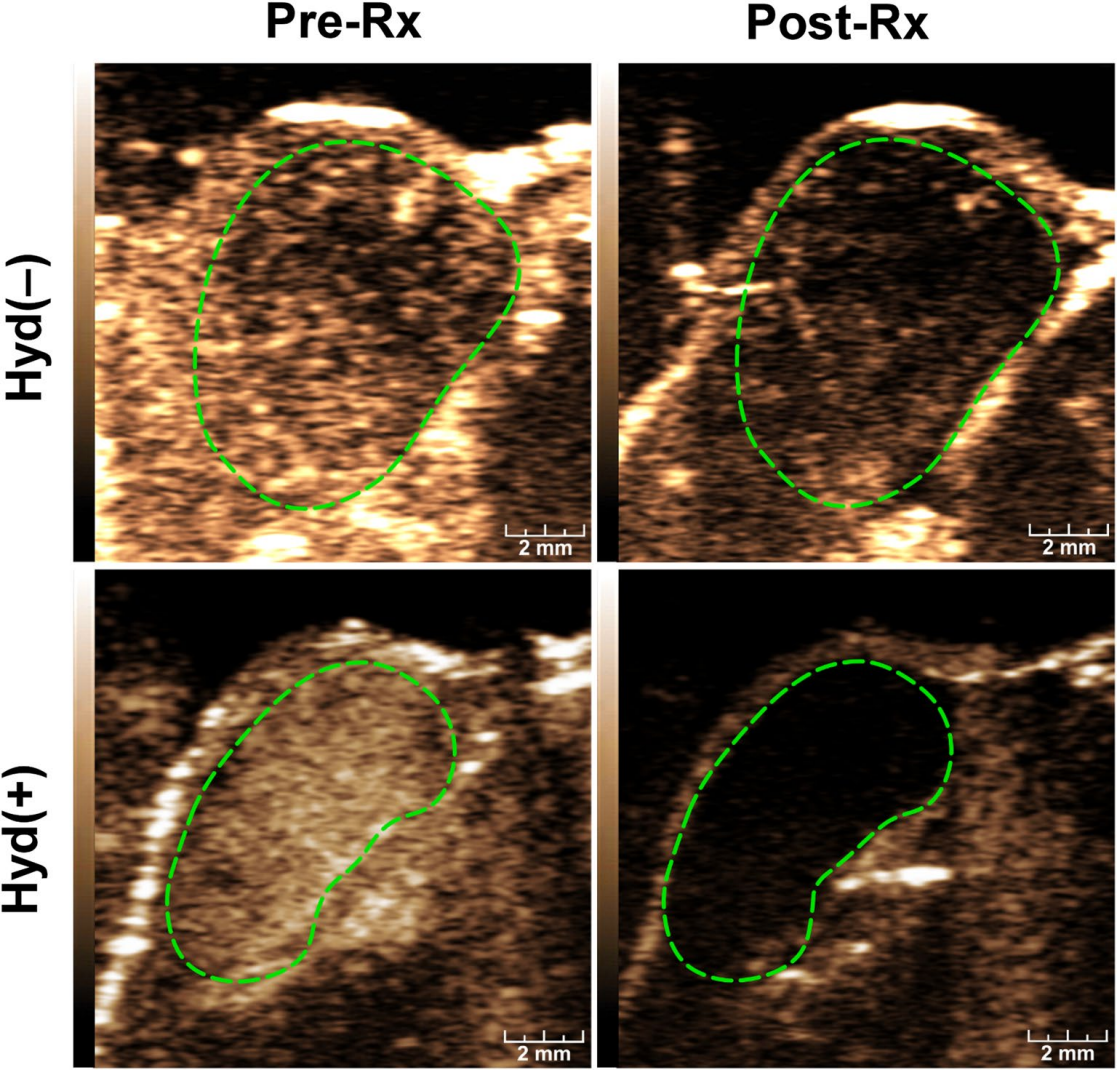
Effects of combined ultrasound and hydralazine treatments on HCC blood flow. Non-linear contrast-enhanced (NLC) images were acquired prior to (Pre-Rx) and following (Post-Rx) ultrasound treatment with [Hyd(+)] or without [Hyd(−)] administration of 5 mg/kg hydralazine; the margins of the tumor were outlined in dotted lines on the grayscale images (not shown) were copied to the NLC image acquired simultaneously. The CEUS images demonstrate that the addition of hydralazine to ultrasound therapy resulted in a further substantial loss in tumor vascularity. Scale bar = 2 mm.
